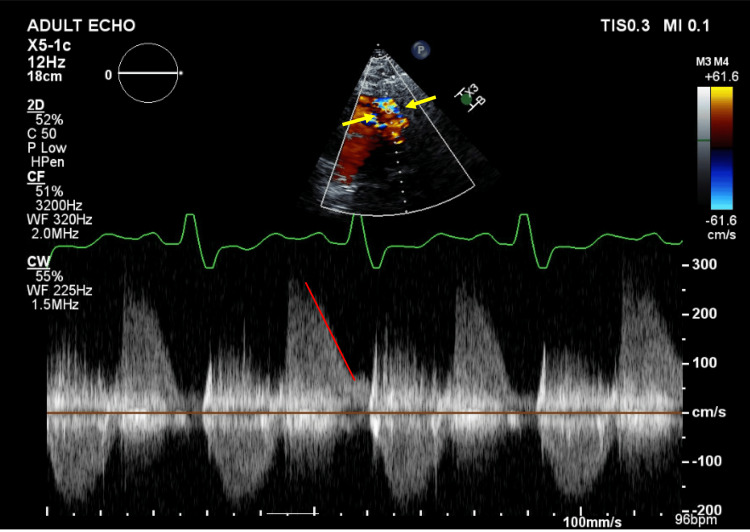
Doppler echocardiogram of the pulmonic valve. The image demonstrates a wide jet width (yellow arrows) and early termination of diastolic regurgitant flow, indicated by a steep slope (red line), indicative of severe pulmonary valve regurgitation. Pressure half-time (PHT) = 111 ms.
